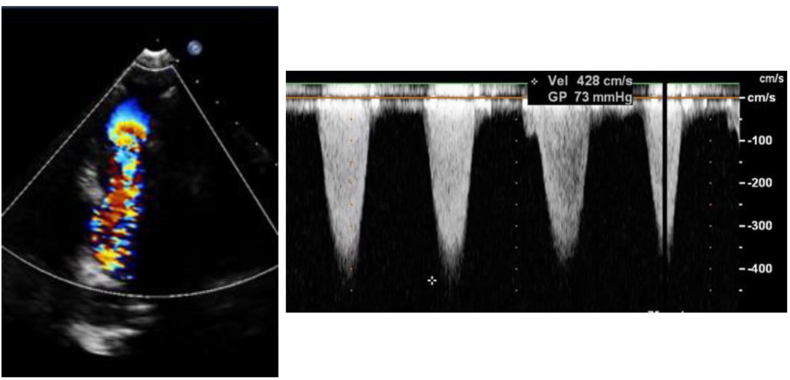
Color doppler and peak transvalvular gradient showing a severe stenosis in a surgically implanted Biopulmonic™ prosthesis in a patient with pulmonary atresia with intact ventricular septum.
